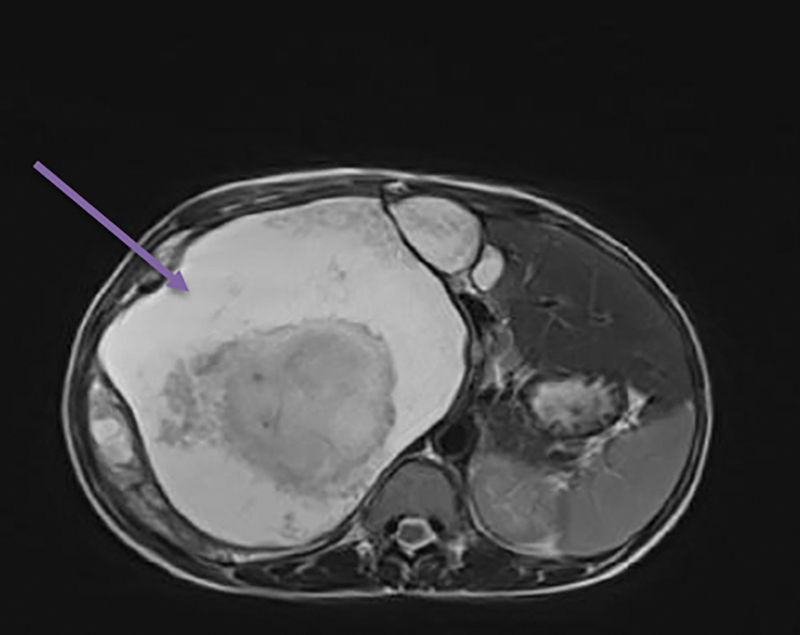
Magnetic resonance imaging cut section showing a large right retroperitoneal mass with variable tissue appearance.
